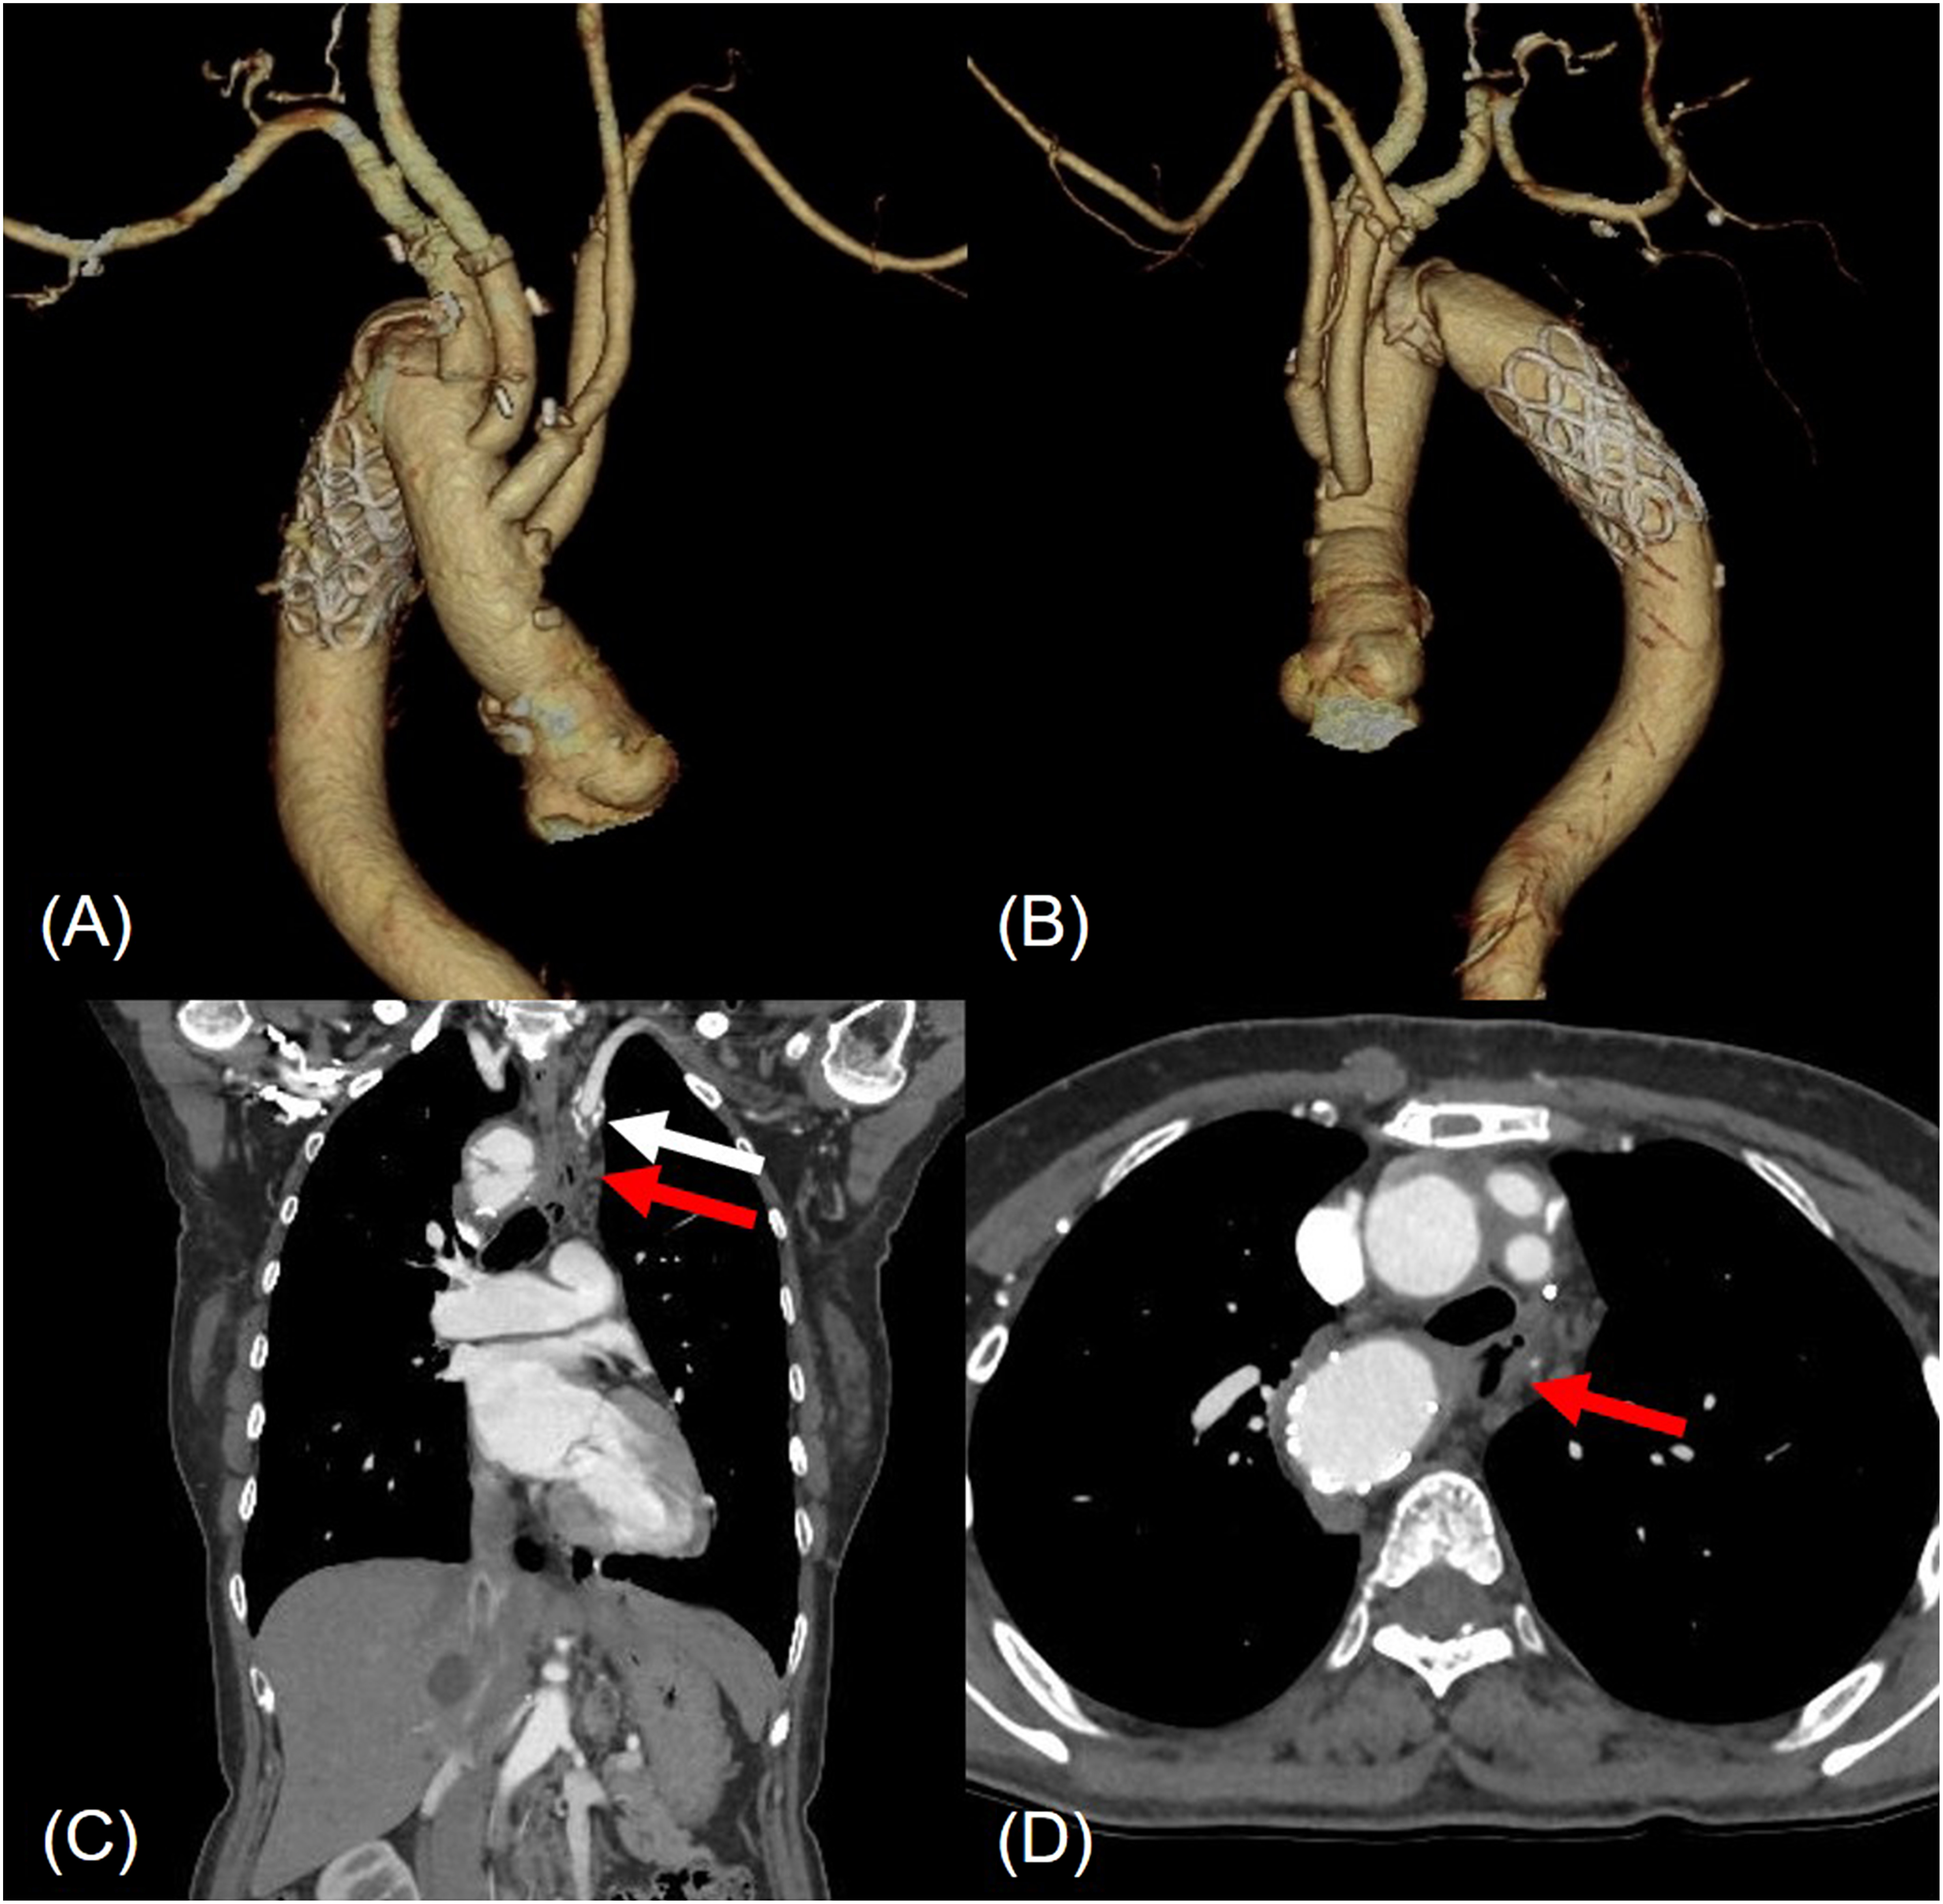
Fig. 3 Postoperative volume rendering computed tomography (CT) image of thoracic aorta. (A) Frontal view. (B) Left lateral posterior view. (C) Coronal CT image of the decompressed esophagus (red arrow) and the reconstructed left subclavian artery (white arrow). (D) Transverse CT image of the decompressed esophagus (red arrow).
